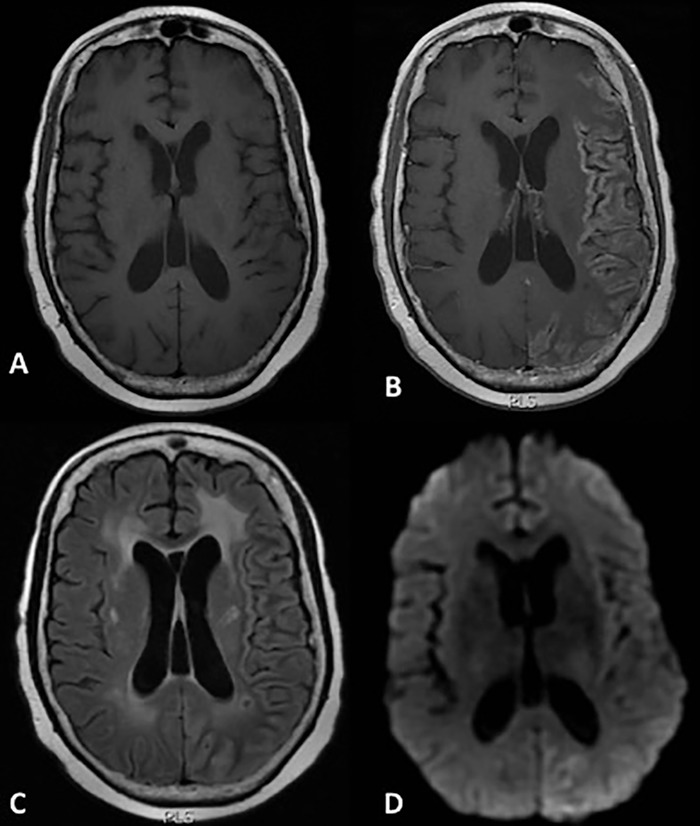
Magnetic resonance imaging on hospitalization day 9. Axial T1-weighted images through the level of the cerebral hemispheres before (A) and after (B) the administration of gadolinium contrast demonstrate diffuse gyral enhancement throughout much of the left cerebral hemisphere with some sparing of the frontal lobe, with corresponding mildly hyperintense signal on a T2/fluid attenuated inversion recovery image (C) and diffusion restriction on diffusion weighted imaging (D).
